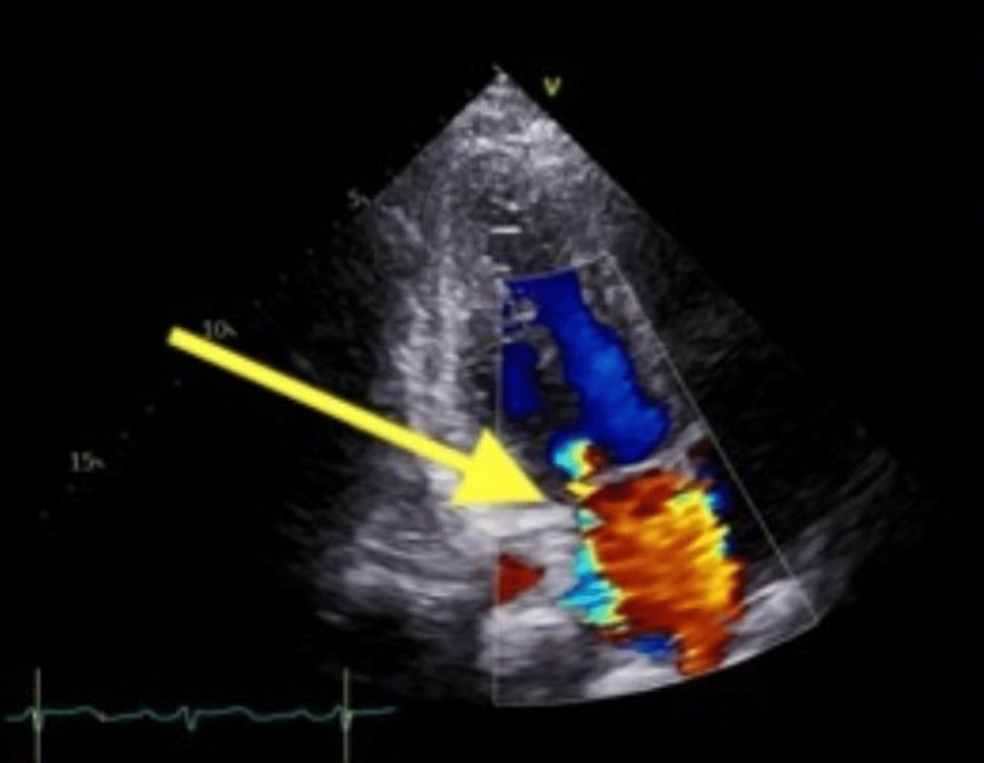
Transthoracic echocardiogram showing severe mitral regurgitation (yellow arrow) with a posteriorly directed jet of the posterior leaflet of the mitral valve
